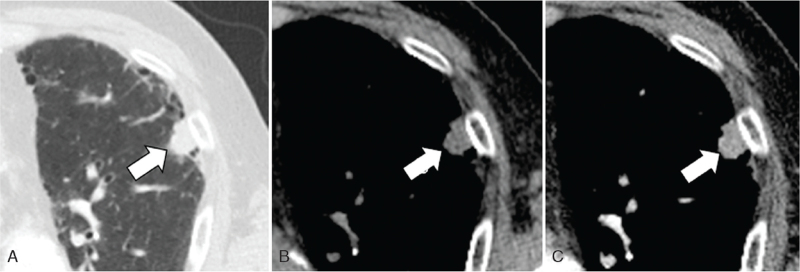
A 74-year-old man with basaloid squamous cell carcinoma of lung. (A) Lung window setting CT scan shows a nodule with lobulated contour (arrow) in left upper lobe subpleural area. Underlying pulmonary fibrosis of usual interstitial pneumonia pattern is noted. Non-enhanced (B) and enhanced (C) CT scans show moderate enhancement of the nodule (arrow) after contrast injection. CT = computed tomography.
